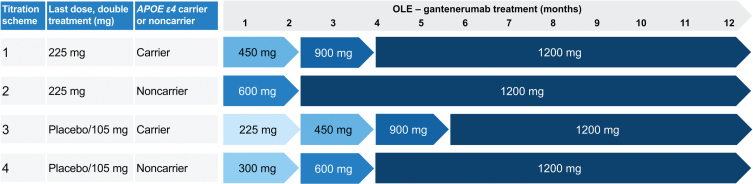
Up-titration regimens for Marguerite RoAD OLE. MRI performed prior to each dose increase and then at regular intervals. Implementation of dosing algorithms in case of ARIA findings. ARIA, amyloid-related imaging abnormalities; *APOE*
